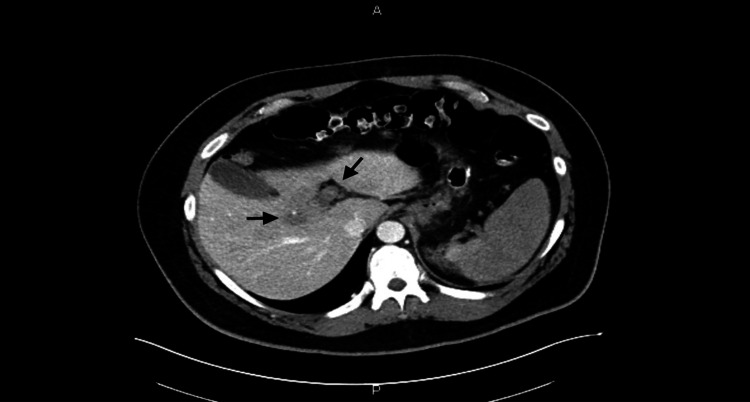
CT with contrast transverse images show nonenhancement of portal venous system consistent with complete thrombosis of portal venous system (Black arrows).
